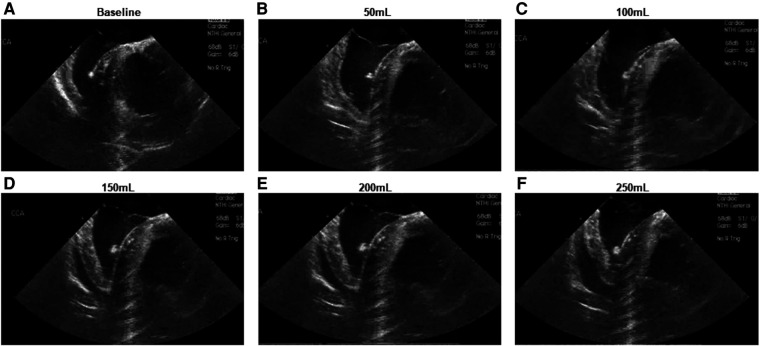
Intracardiac echocardiographic appearance of developing pericardial effusion. (A) Baseline appearance (B–F) Appearance following infusion of (B) 50 ml (C) 100 ml (D) 150 ml (E) 200 ml (F) 250 ml.
